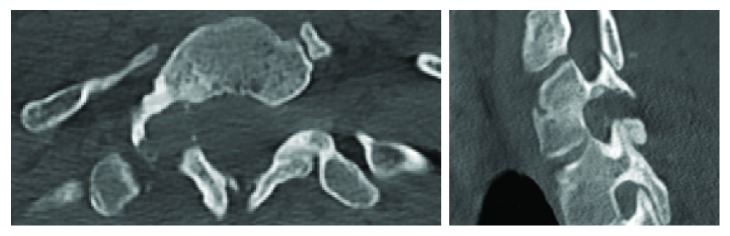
Postoperative spine CT scan after one week. The images confirmed the complete resection of the lesion, without destabilizing the vertebral column.
